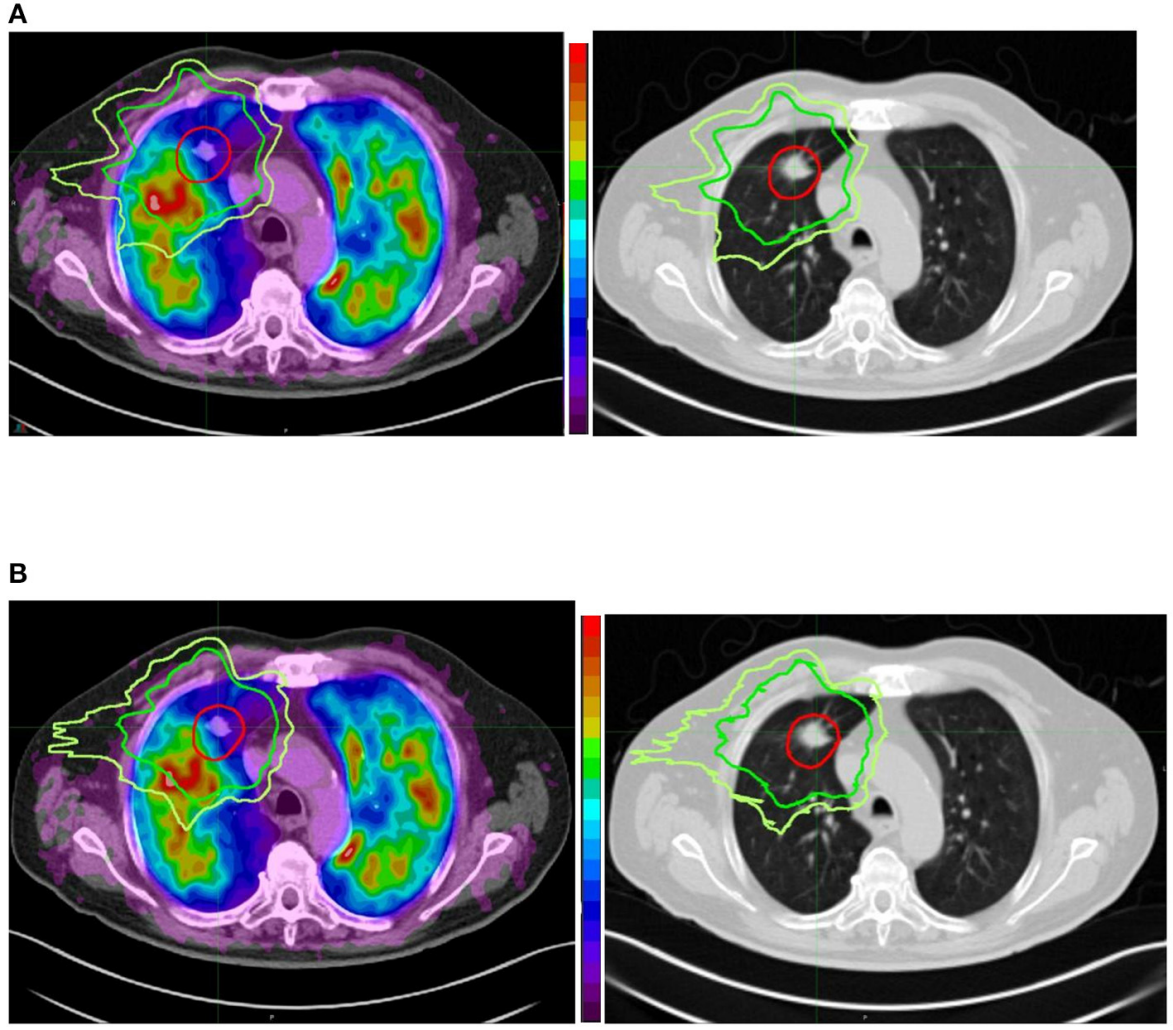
Comparison between conventional (anatomically based) planning (A) and functional lung avoidance planning guided by ^68^Ga Q PET/CT (B) in a patient treated for a lung tumor by stereotactic body radiation therapy. Both planning show optimal coverage (red isodose line) of the tumor but the functional planning shows a decrease of the dose (20 Gy isodose in green and 16 Gy isodose in yellow) to the functional lung (in red the most functional areas and in blue the least functional areas). We can also see that on the corresponding anatomical images we cannot see any difference between the functional and non-functional areas.
